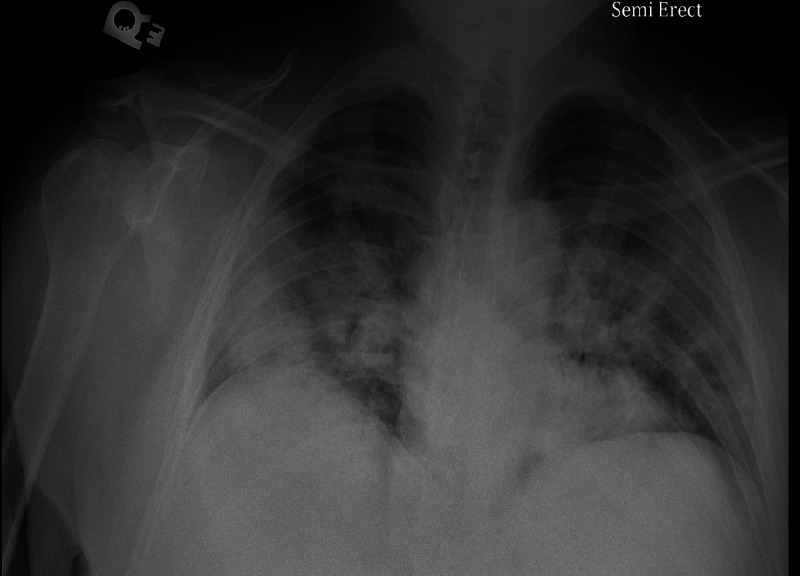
Portable chest X-ray significant for bilateral patchy airspace opacities, concerning for pneumonia
